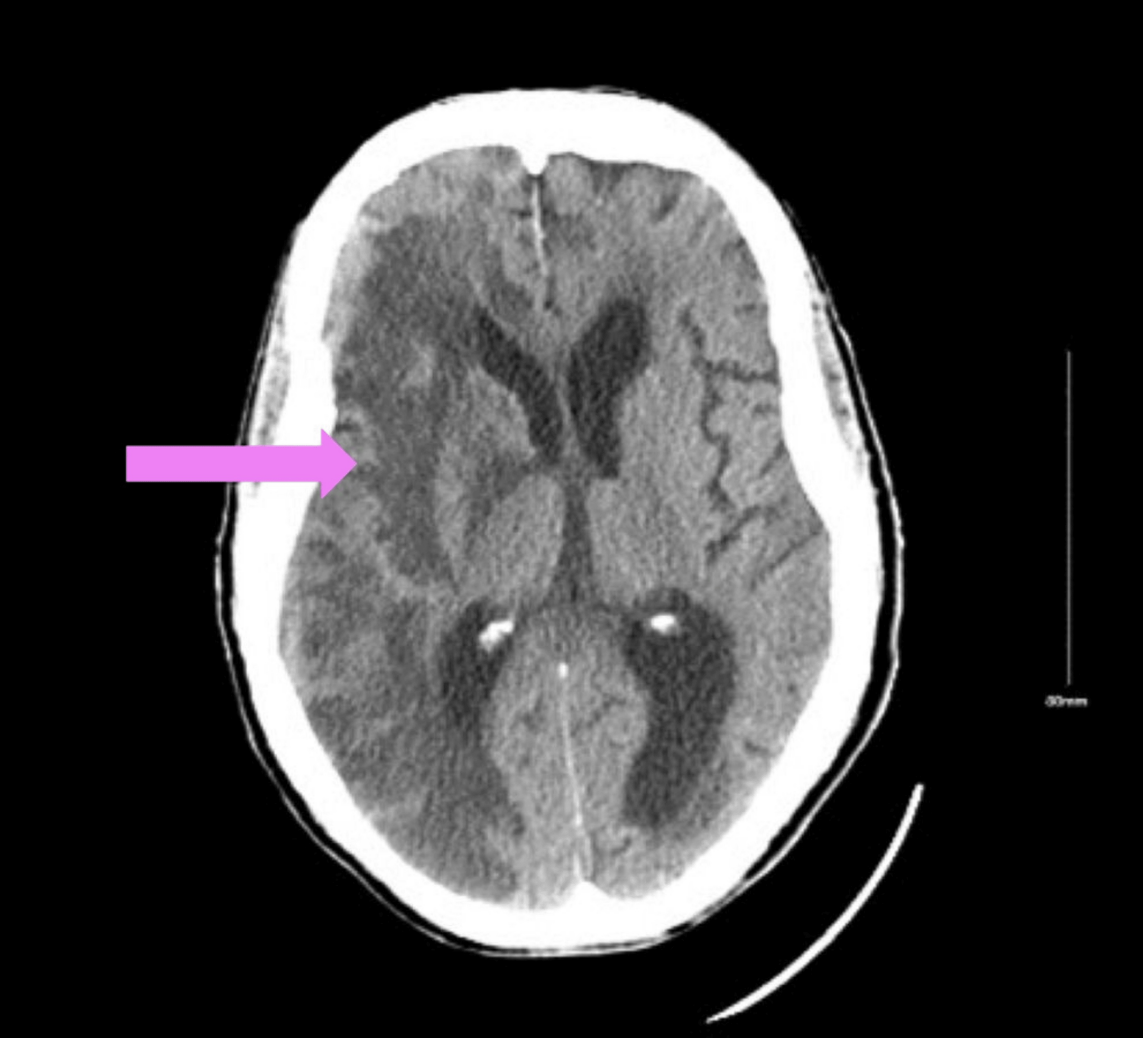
Computed tomography head shows extensive region of infarcted brain parenchyma in the right anterior and middle cerebral artery territories, secondary to right internal carotid artery occlusion.
